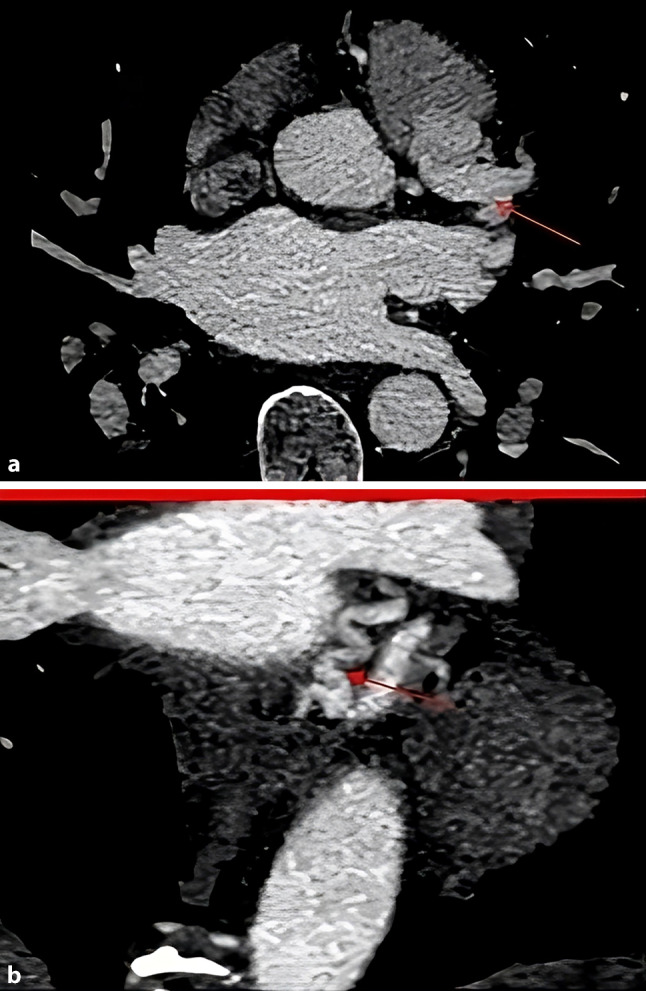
a Computed tomography angiography showed an anomalous origin of the left coronary artery (*LCA*, *red arrow*) from the pulmonary artery (*PA*), b an ectatic and tortuous LCA (*red arrow*)
